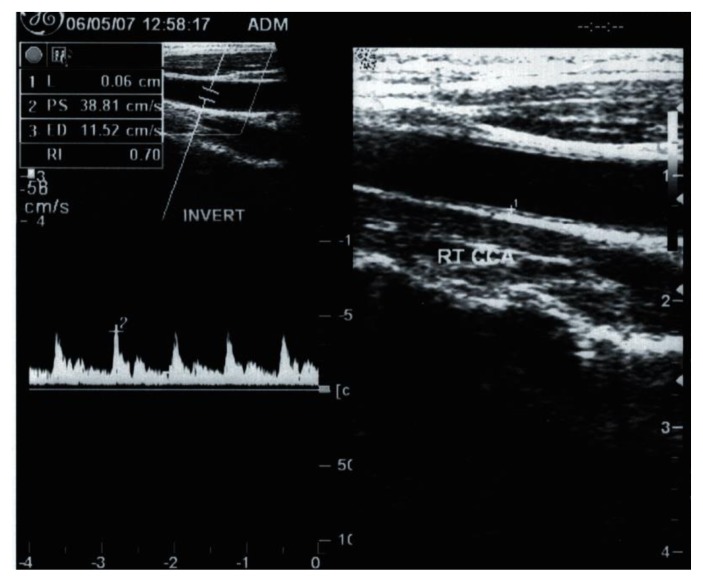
Long-axis view and Doppler spectrum of the right CCA showing increased intima-media thickness of 0.6-mm in an 11-year-old child with thalassemia.
